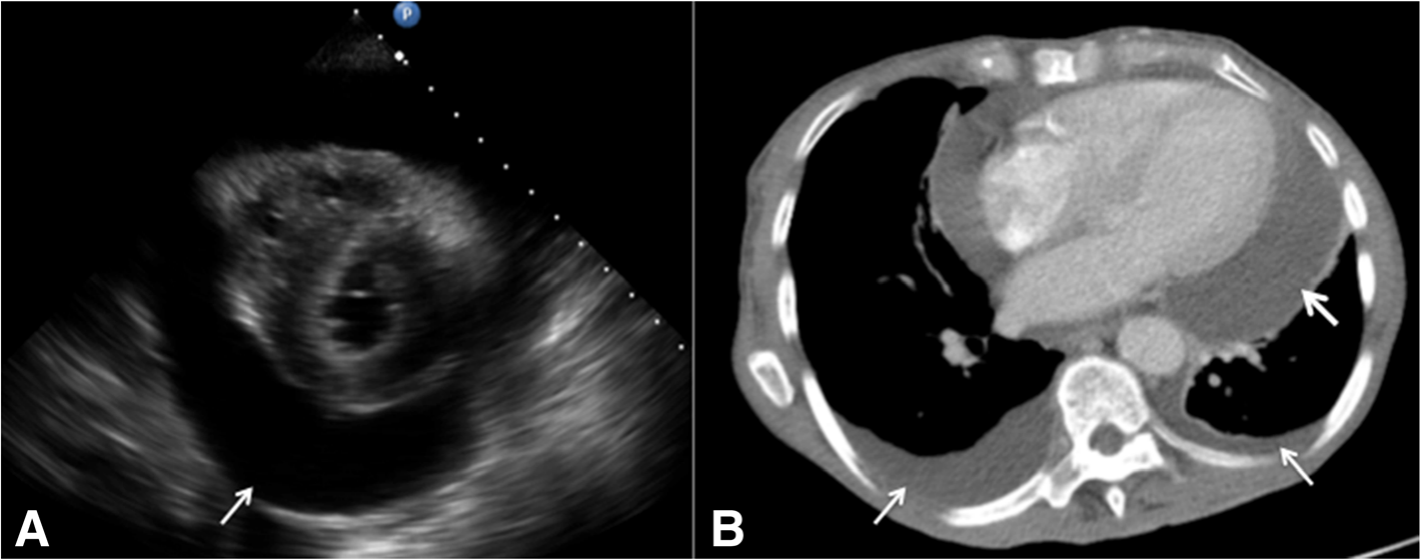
A 72-year-old man with history of lung cancer presenting pericardial effusion/cardiac tamponade. (A) Echocardiogram demonstrating hypoechoic pericardiac fluid (arrow) and through transmission. (B) Axial computed tomographic slice of the thorax obtained in a mediastinal window after intravenous contrast administration showing a large pericardial effusion (thick arrow) and small bilateral pleural effusions (thin arrows).
